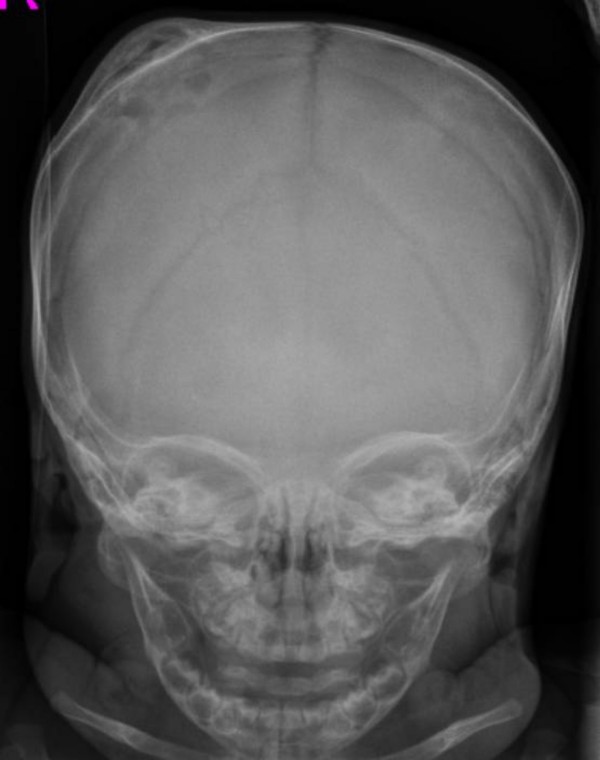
Anteroposterior skull radiographs showed a multicystic lesion with slightly sclerotic margin in the right parietal bone, associated with significant outward bulging of the outer table without destruction of the inner table in tangential view of the right parietal bone.
